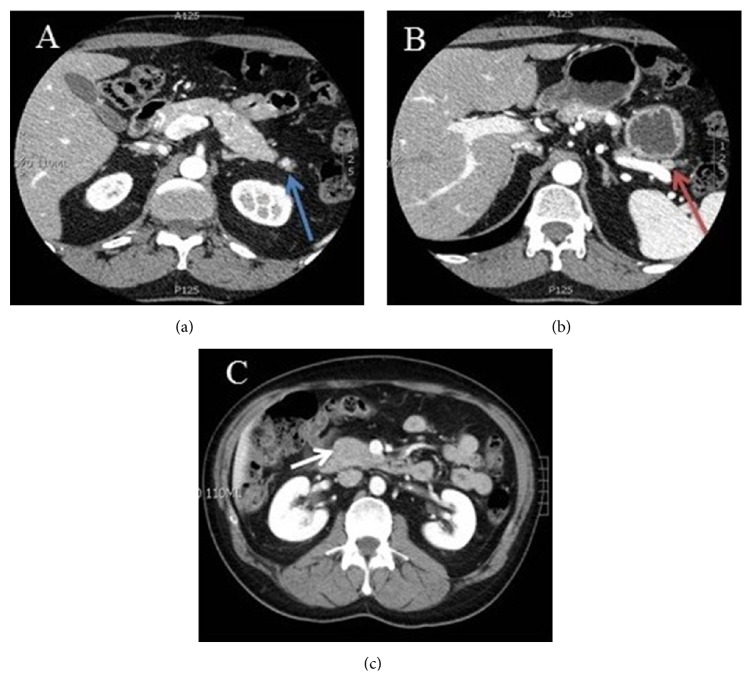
CT of the pancreas showing three hyperenhancing lesions which have remained stable 38 months after initiating octreotide. (a) Stable 4 mm tumor in the inferior aspect of the pancreatic tail (blue arrow). (b) Stable lesion in the superior aspect of the pancreatic tail (red arrow). (c) Stable lesion in the pancreatic head.
